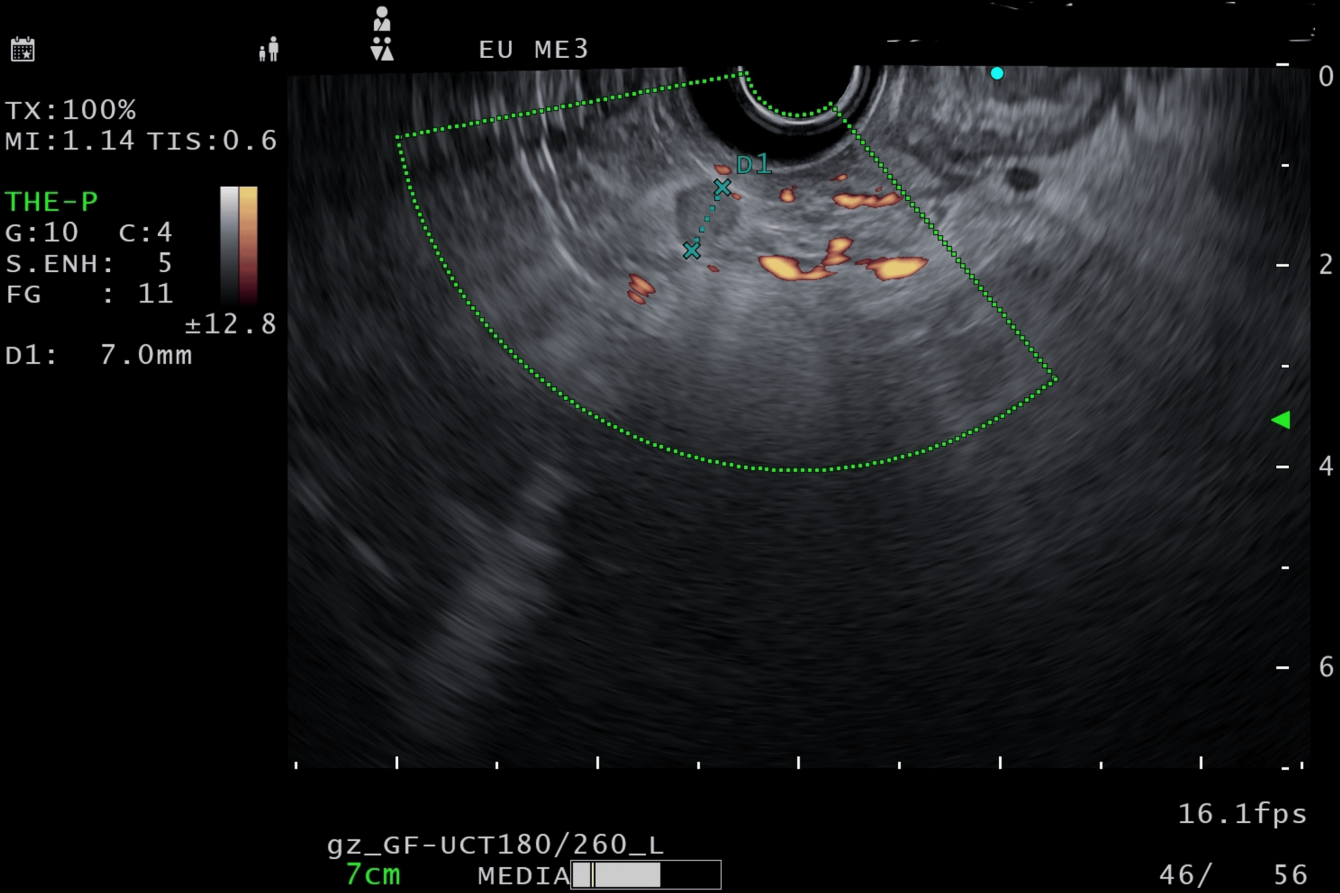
EUS localization of metastatic paraduodenal lymph nodes EUS: Endoscopic ultrasound
